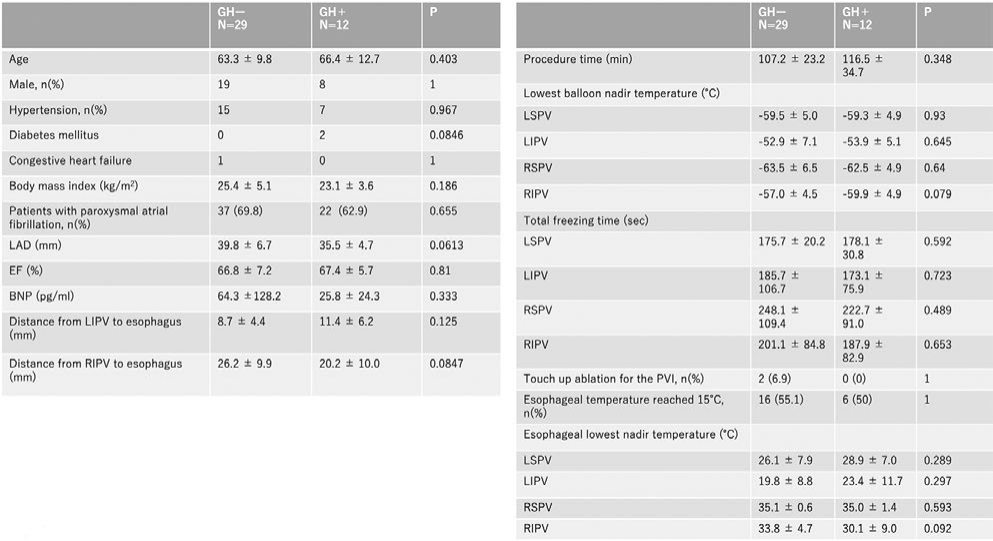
## COMPARATIVE IMPACT OF DIFFERENT ENERGY SOURCES ON ATRIAL FUNCTION AFTER PULMONARY VEIN ISOLATION: A SINGLE‐CENTER RETROSPECTIVE ANALYSIS
